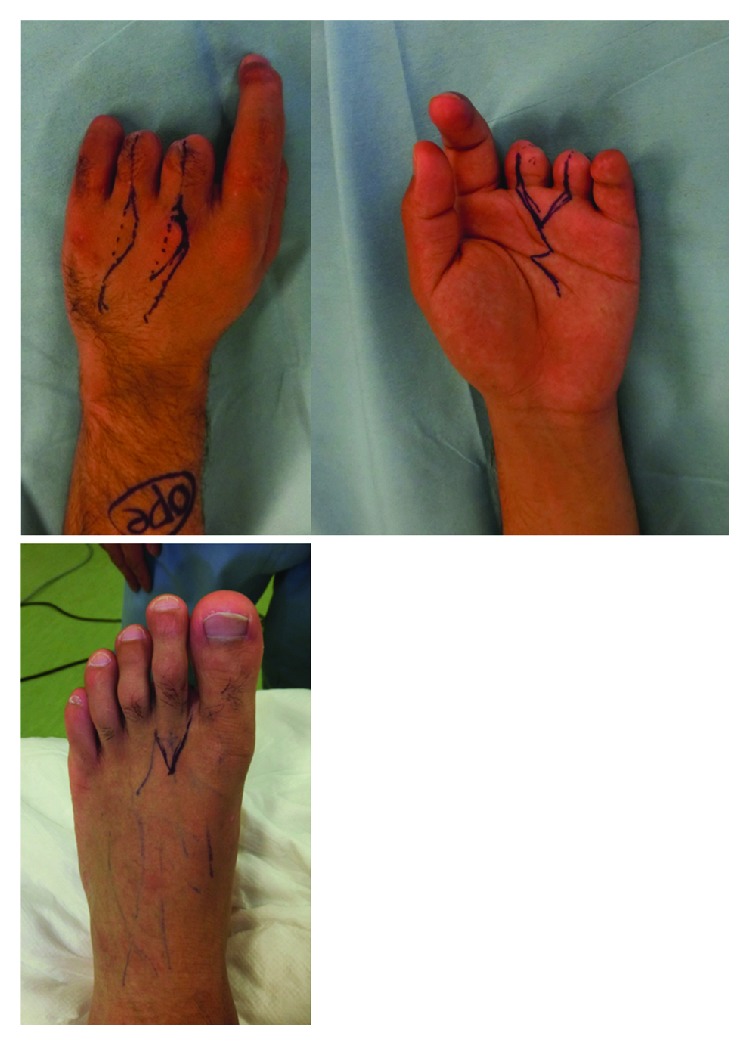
Design of the hand. We confirmed the dorsal metacarpal vein with ultrasonography.
